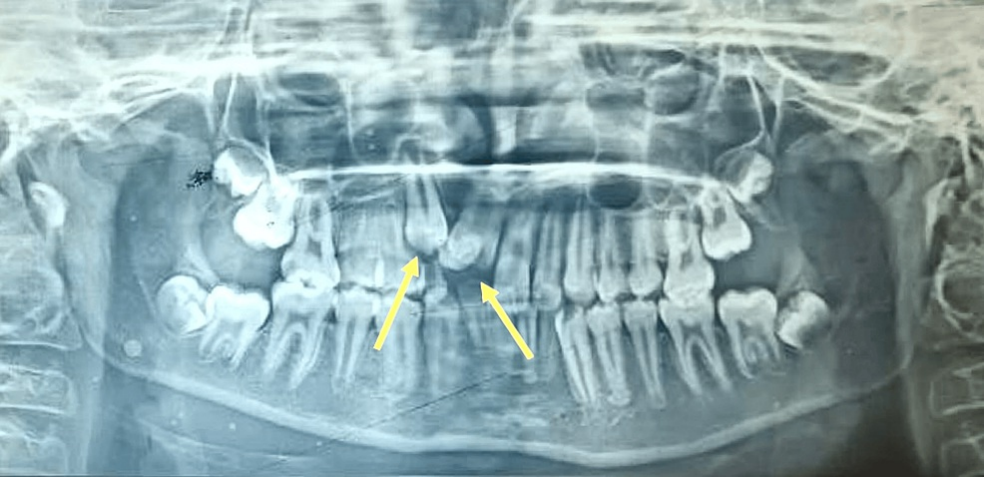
Pre-treatment orthopantomogram (OPG) OPG reveals impacted 11, 12, and 13 along with over-retained 53
